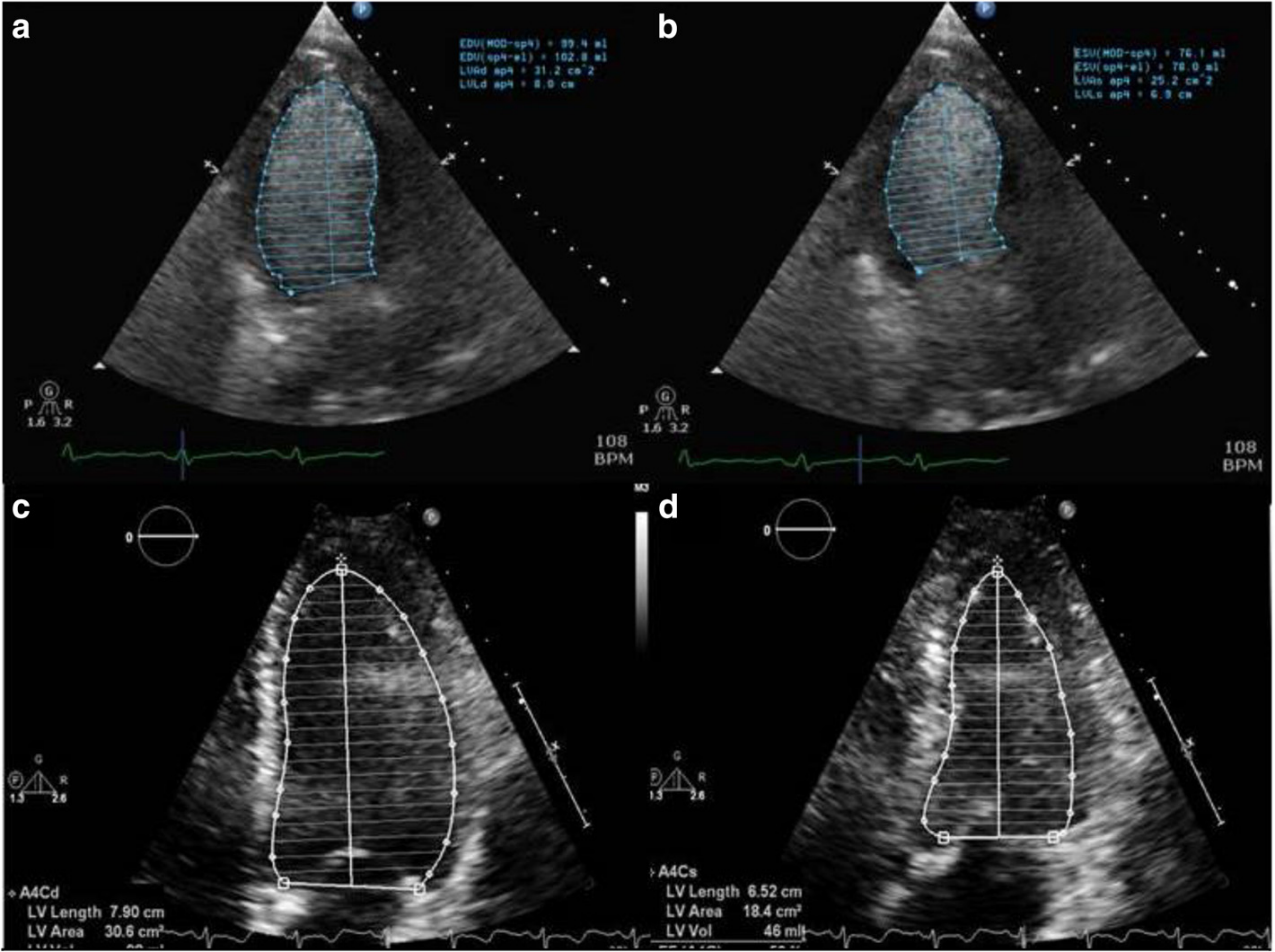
Transthoracic echocardiogram at Day 1 and Day 7, Apical four chamber view from day 1 transthoracic echocardiogram (TTE) with IV contrast at end-diastole a and end-systole b demonstrates depressed left ventricular (LV) ejection fraction estimated at 26 %. Apical four chamber view from day 7 TTE at end-diastole c and end-systole d demonstrates normalized LV ejection fraction
